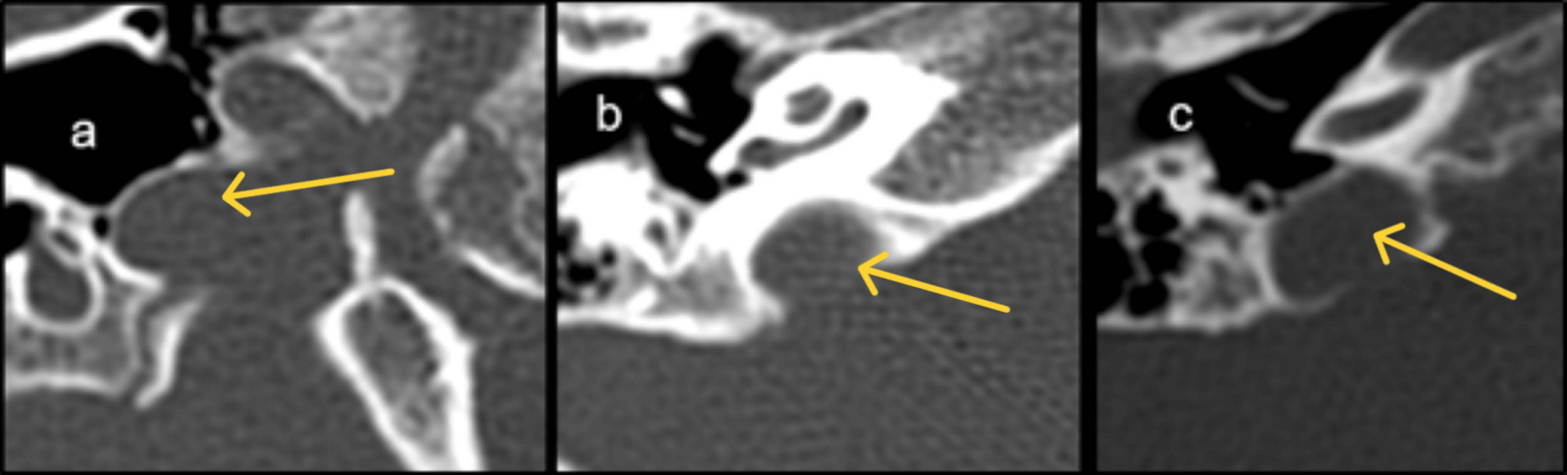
(a)-(c) Axial images of HRCT temporal bone demonstrating the position of the jugular bulb. (a) Normal, (b) high riding, and (c) high-riding with focal dehiscence. HRCT: high-resolution computed tomography.
